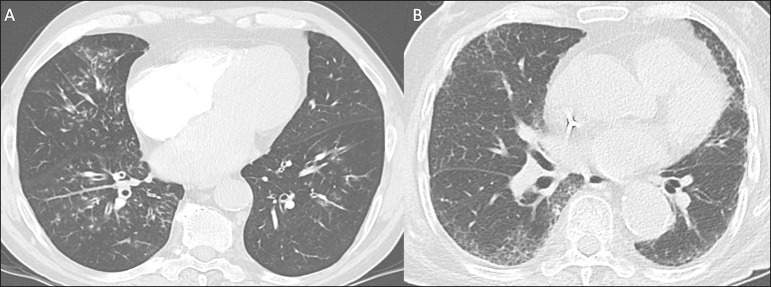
Examples of atypical findings for COVID-19 on chest CT. A: Small nodules with centrilobular “tree-in-bud” distribution in the right lung; RT-PCR test was positive for enterovirus. B: Typical findings of usual interstitial pneumonia in a patient with multiple myeloma.
